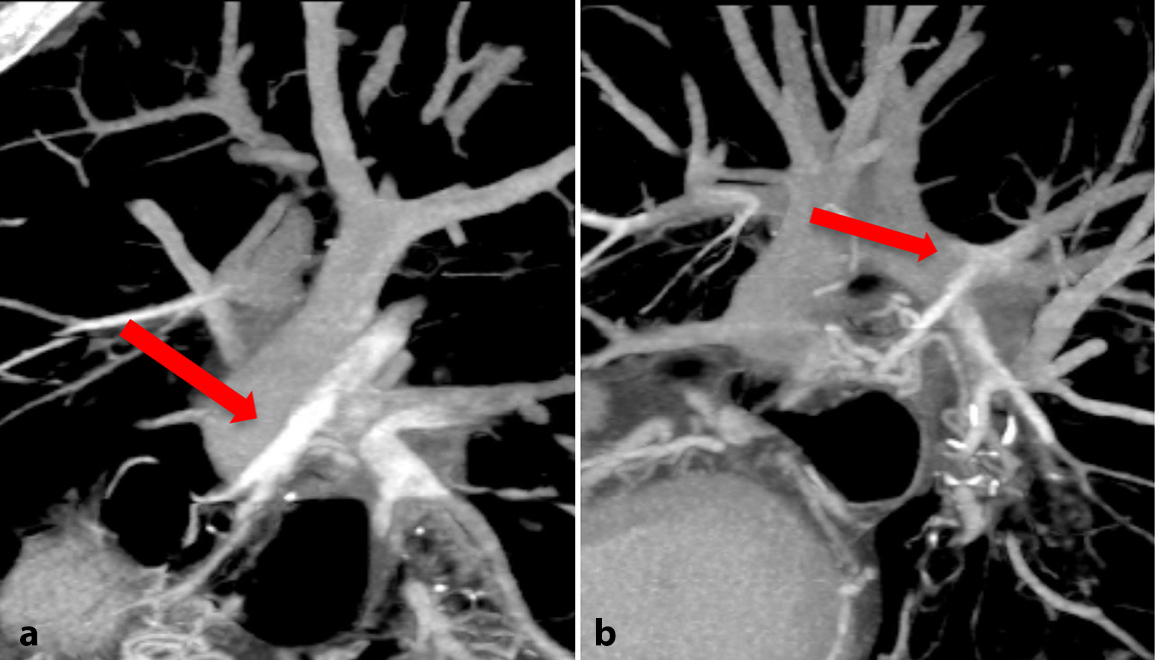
(a) Curved reformat images from CT aortic angiogram showing heavily opacified bronchial arteries (red arrow) anastomosing with pulmonary arteries. (b) A blush of contrast is seen spilling into the relative negative contrast in the pulmonary arterial circulation (red arrow).
